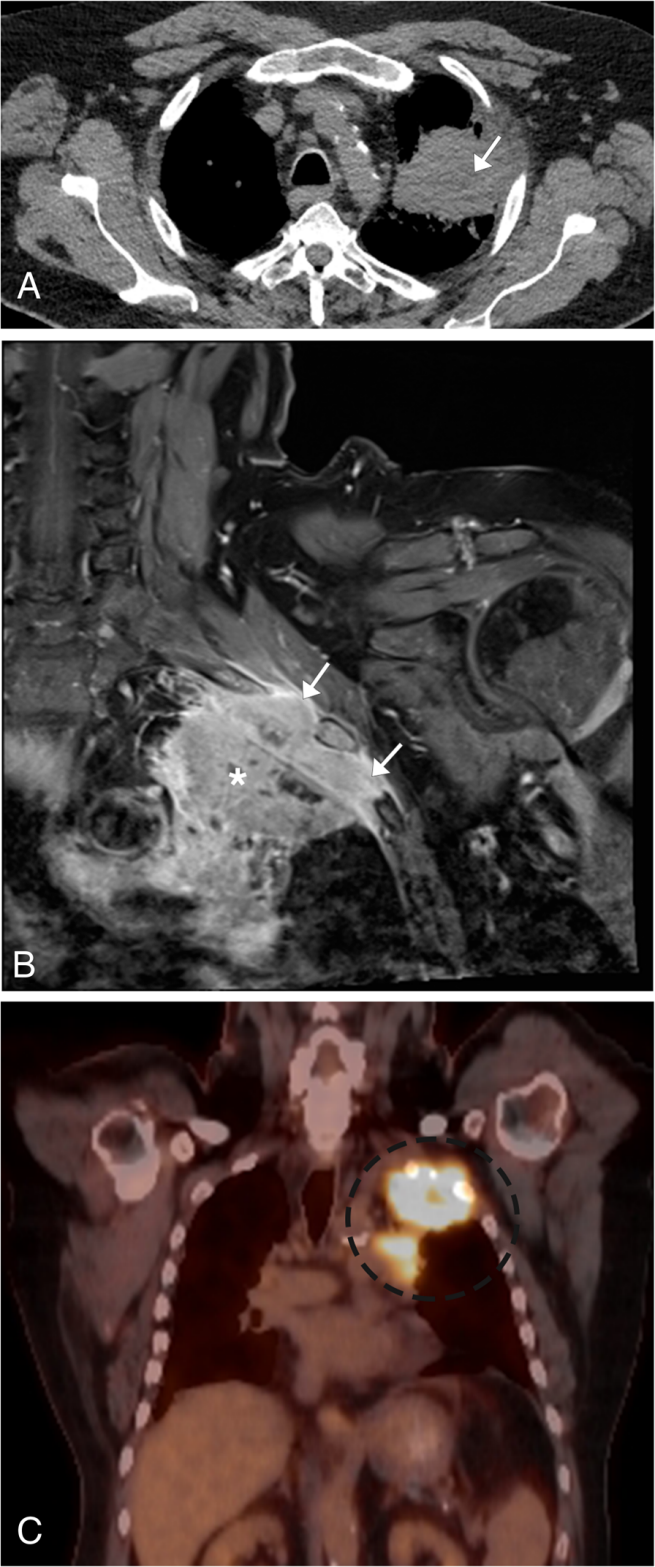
Pancoast Tumor. a Axial non-contrast CT of the chest demonstrates a large solid irregular mass in the left lung apex (arrow). b Coronal T1WI MR post contrast shows the enhancing mass (*) invading into the chest wall with involvement of the adjacent first to third left ribs (arrows). c Coronal FDG-PET/CT image demonstrates that the left lung apex mass is avidly hypermetabolic (dashed circle)
